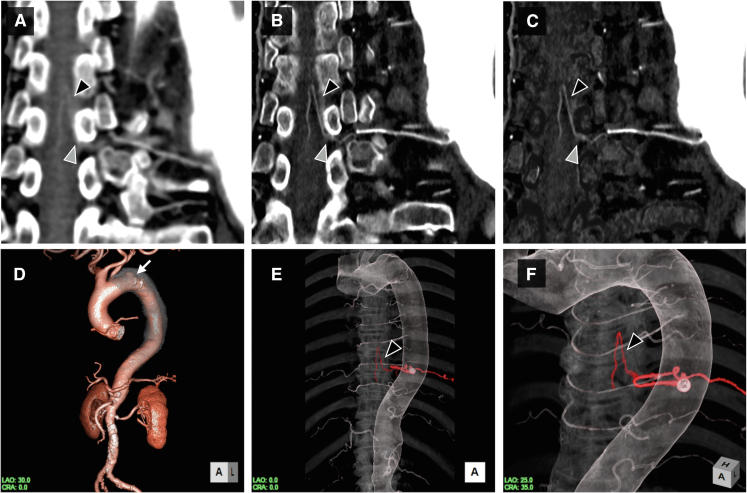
Representative case of concordant Adamkiewicz artery (AKA) identification in standard computed tomography angiography (CTA) and AKA-specific computed tomography (AKA-CTA) of an 85-year-old woman with aortic dissection. Curved multiplanar reformatted images of CTA (A), AKA-CTA (B), and bone-subtracted AKA-CTA (C), volume-rendered images of CTA (D), and AKA-CTA (E-F) are shown. The hairpin-shaped vasculature (*black arrowhead*) is the AKA, arising from the left T9th segmental artery. The continuity of the AKA in the intercostal foramen in standard CTA is equivocal (*gray arrowhead*) (A) but definitive in AKA-CTA (B, C). An ulcer-like projection at the proximal descending artery is indicated by a *white arrow* (D).
